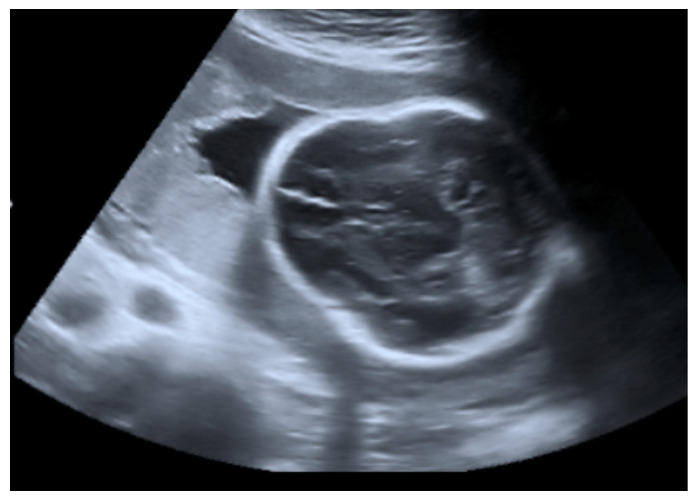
Transabdominal fetal ultrasound scan image including an axial view of the fetal head in the transthalamic plane. A deformed skull contour was observed.
